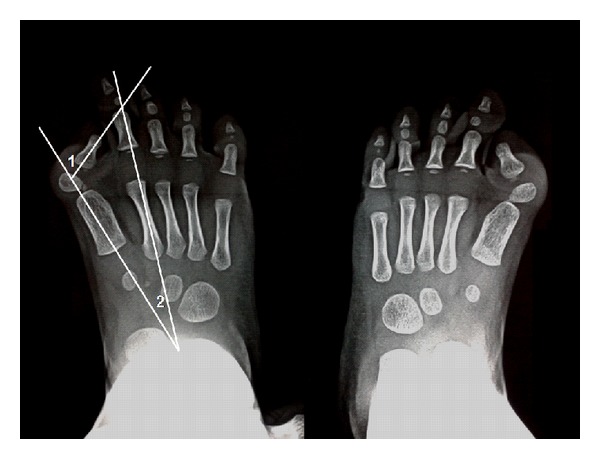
X-ray for the baby's feet showing rudimentary proximal phalanges, hallux valgus deformities with hallux valgus angle (HVA); angle (1) 65° and intermetatarsal angle (IMA); angle (2) 20°.
